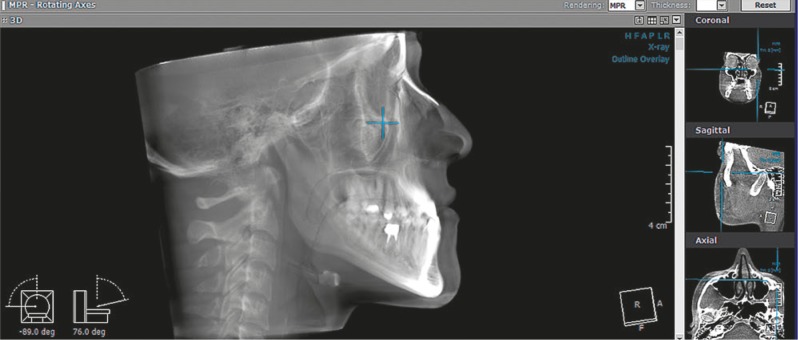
Example of CBCT cephalogram used in this study.
